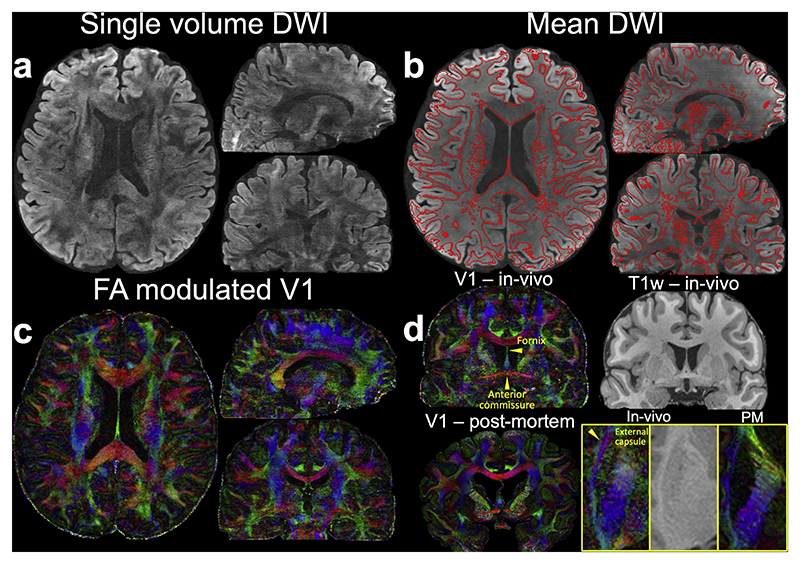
Data from 3T 0.65 mm protocol. (a) In-vivo diffusion data (*b* = 1000 s/mm^2^) including diffusion-weighted image (DWI) along (–0.45, 0.83, –0.32), (b) 6-direction mean DWI with gray-white matter boundary derived from the T1w image using overlayed, (c) fractional anisotropy (FA) modulated V1 at 0.65 mm isotropic resolution, (d) a representative sagittal view of the FA modulated V1 (V1 – in-vivo) alongside the T1-weighted image (T1w – in-vivo), highlighting key structures including the fornix, external capsule, and anterior commissure are presented. For comparison, post-mortem (PM) data (V1 – post-mortem) from previous studies (Tendler et al., 2022) are shown. Enlarged views of the external capsule from an axial plane are provided for both in-vivo and post-mortem data (d).
